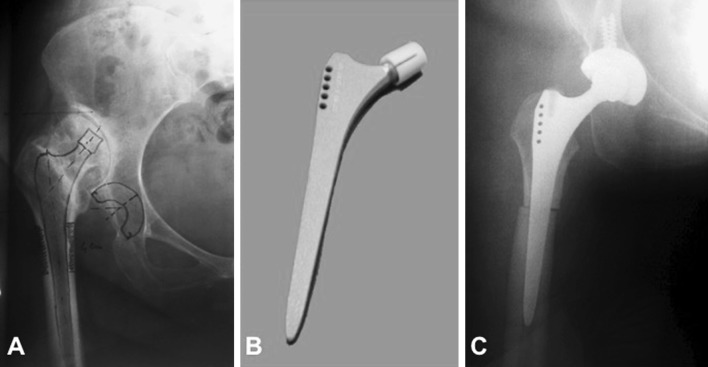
39-year-old male patient with unilateral Crowe type IV DDH. a Pre-operative planning with cementless THA and transverse shortening osteotomy. b Quadrangular cross-section CSR Japan stem (Sulzer^®^, Switzerland). c Post-operative X-ray
